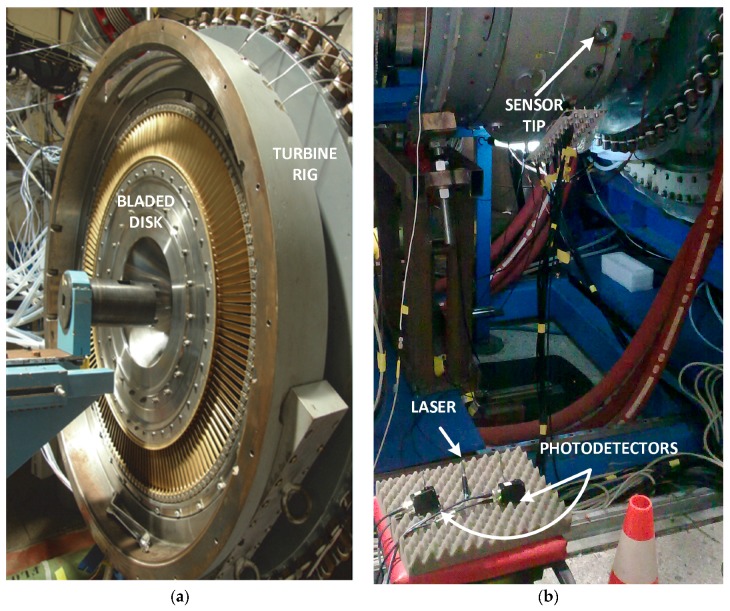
(a) Compressor disk with 146 blades in the turbine rig; (b) sensor test at the Aeronautical Technology Center (CTA).
